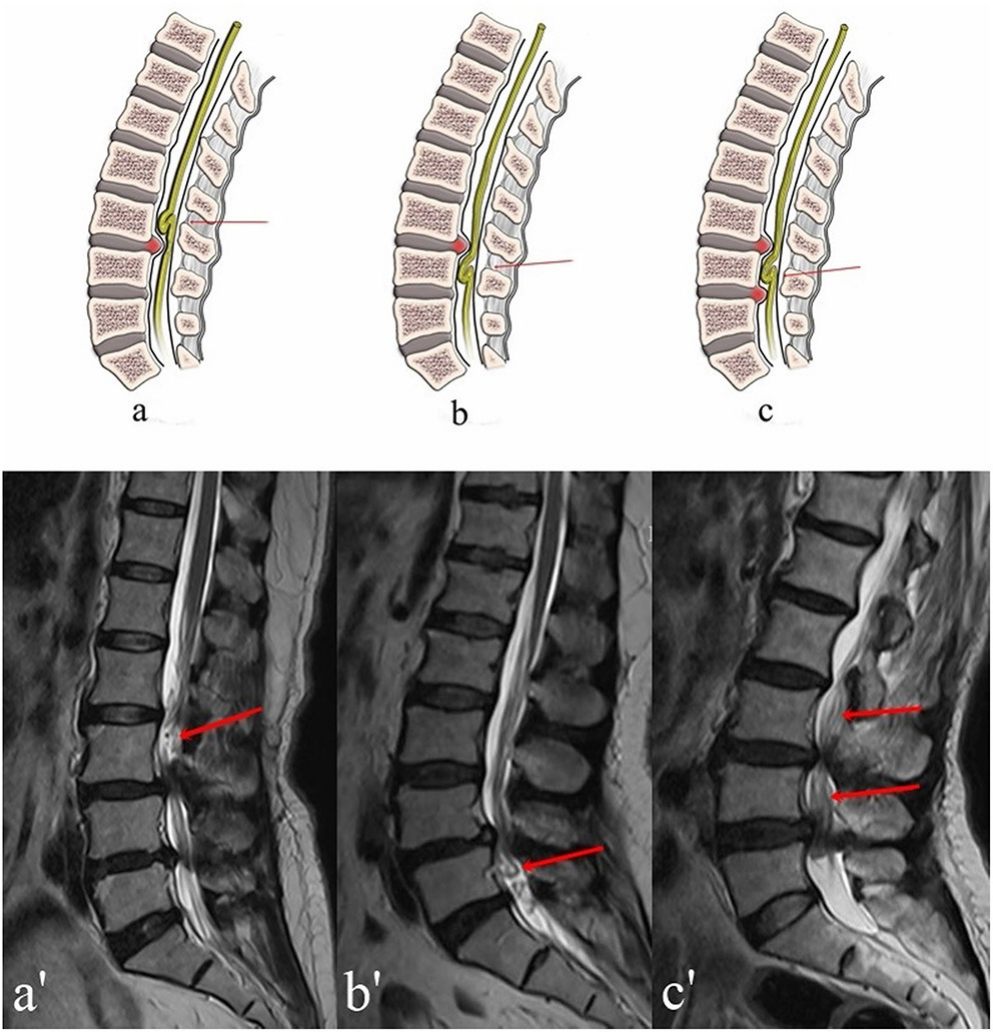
Three possible different location of RNRs with corresponding MRI images. (A,A′) Upper: redundant nerve roots (red arrow) above the level of maximal stenosis. (B,B′) Lower: redundant nerve roots (red arrow) below the level of maximal stenosis. (C,C′) Intermediate: redundant nerve roots (red arrows) between two levels with severe stenosis.
